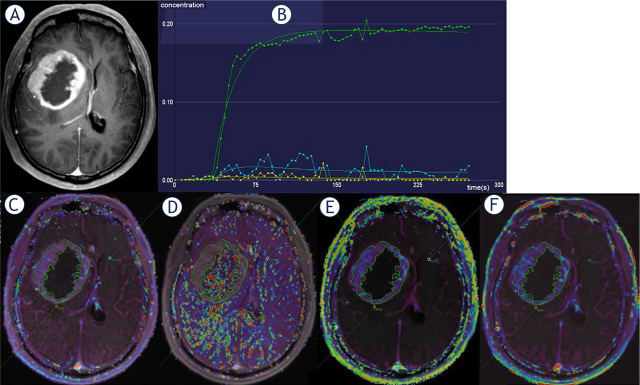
Representative ROI delineations. A 53-year-old man was diagnosed with glioblastoma, IDH-wildtype. (A) contrastenhanced T1-weighted image (T1WI-CE); (B) the time-signal intensity curve; (C) the transfer constant (*ktrans*) image; (D) rate constant (*kep*) image; (E) extravascular extracellular volume fraction (*ve*) image; (F) initial area under the curve in the first 60 s (*iauc*) image. On images, B-F, ROI 1 marked green represented tumor parenchyma, ROI 2 marked yellow represented the peripheral zones, and ROI 3 marked blue-turquoise represented contralateral normal-appearing brain tissues.
